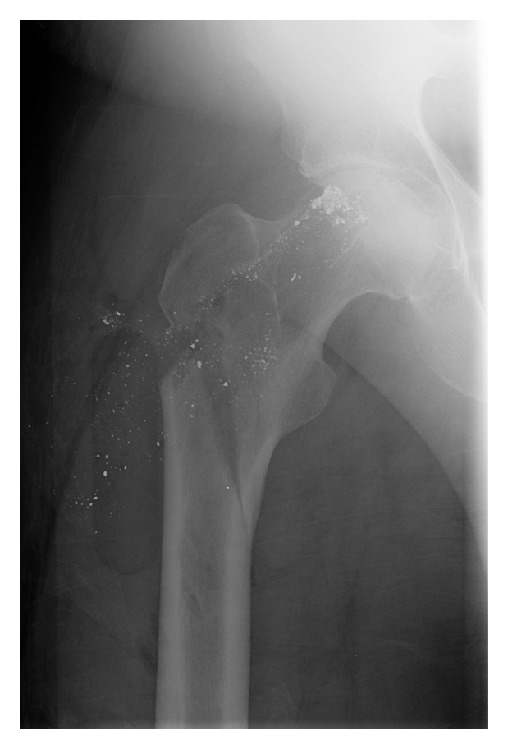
Postoperative day 1 right hip radiograph demonstrating a subtrochanteric femur fracture after the patient rolled in bed and heard a “crack.”
